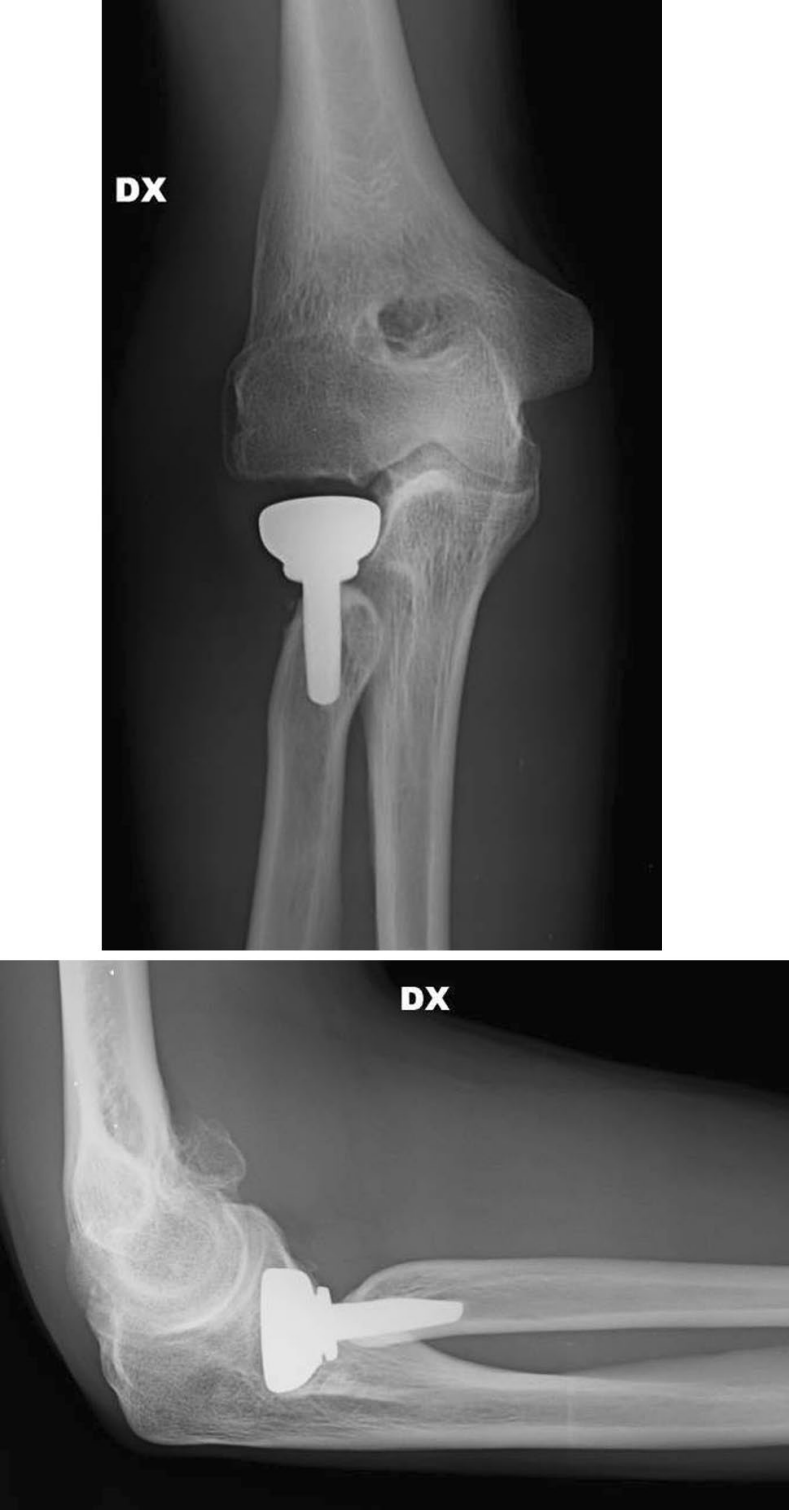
Five-year X-ray follow-up with good position of implant and stress shielding radial neck resorption
